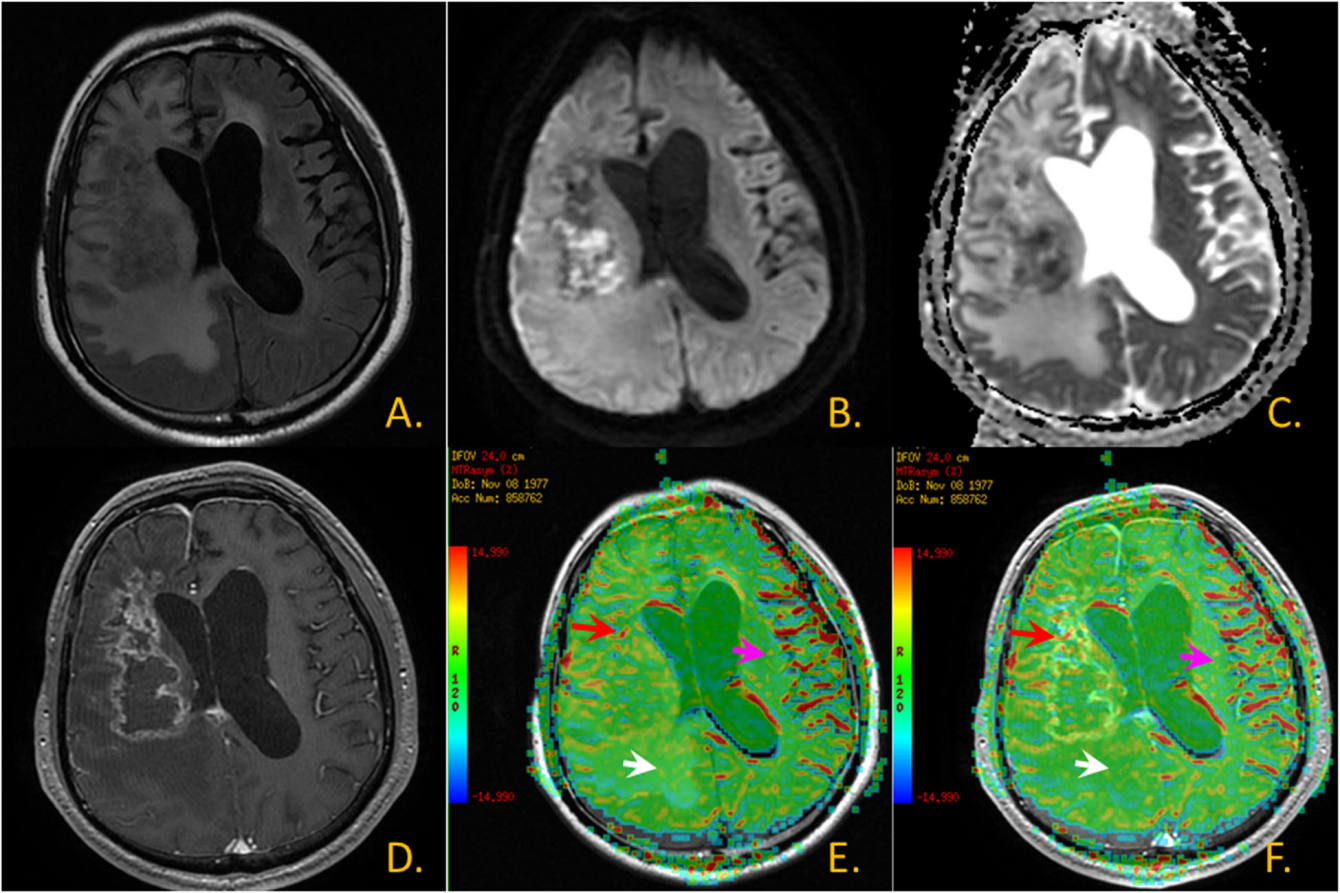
Glioma images. a. T2 FLAIR image; b. DWI; c. ADC map; d. T1WI + C; e. CEST MTR map and T2 FLAIR image fusion: The red arrow area is shown as lesion-parenchymal areas, The white arrow area is shown as edema areas and The pink arrow area is shown as non-focus areas; f. CEST MTR map and T1WI + C fusion: The red arrow area is shown as lesion-parenchymal areas, The white arrow area is shown as edema areas and The pink arrow area is shown as non-focus areas
